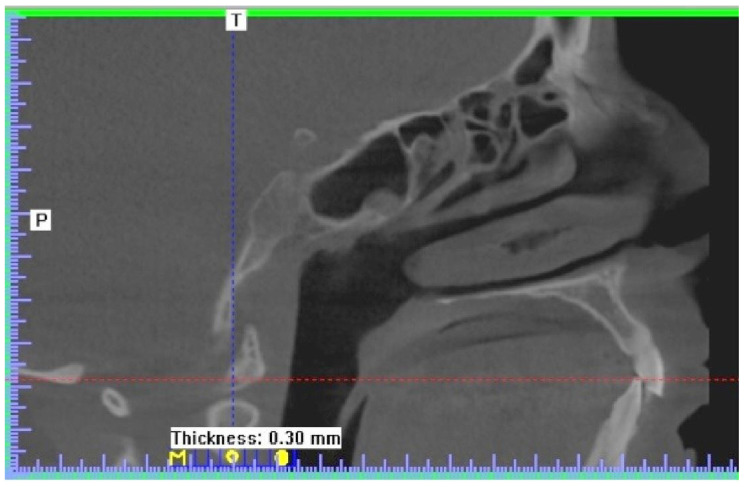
Ethmoid bulla and the sphenoid sinus—status after a series of 30 physical therapy sessions. (CBCT scan).
